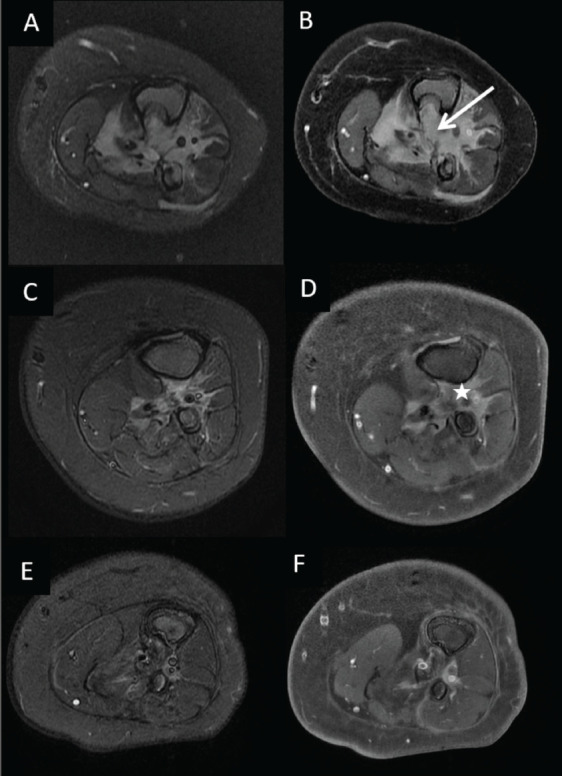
Pre-chemotherapy; (a): axial T2W (fat saturated) and (b): contrast-enhanced T1W (fat-saturated) MRI images showing Ill-defined homogenously enhancing mass lesion in the Interosseous space (arrow) causing erosion of tibia and fibula. (c, d): Corresponding post-chemotherapy MR images showing significant reduction of the mass lesion with minimal enhancing residual soft tissue lesion at the end of 3 months of larotrectinib (Asterix) and (e, f) complete response after 8 months of therapy.
